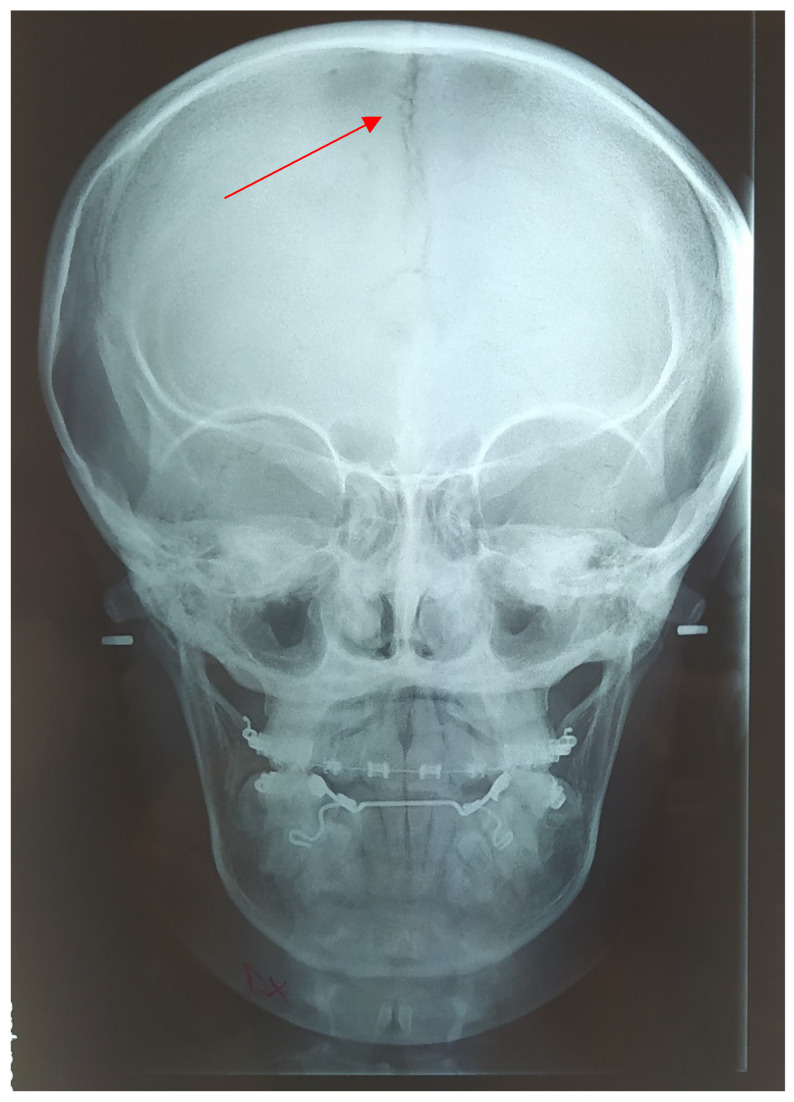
Teleradiography antero-posterior X-ray in 2021, presence of open cranial sutures (red arrow).
